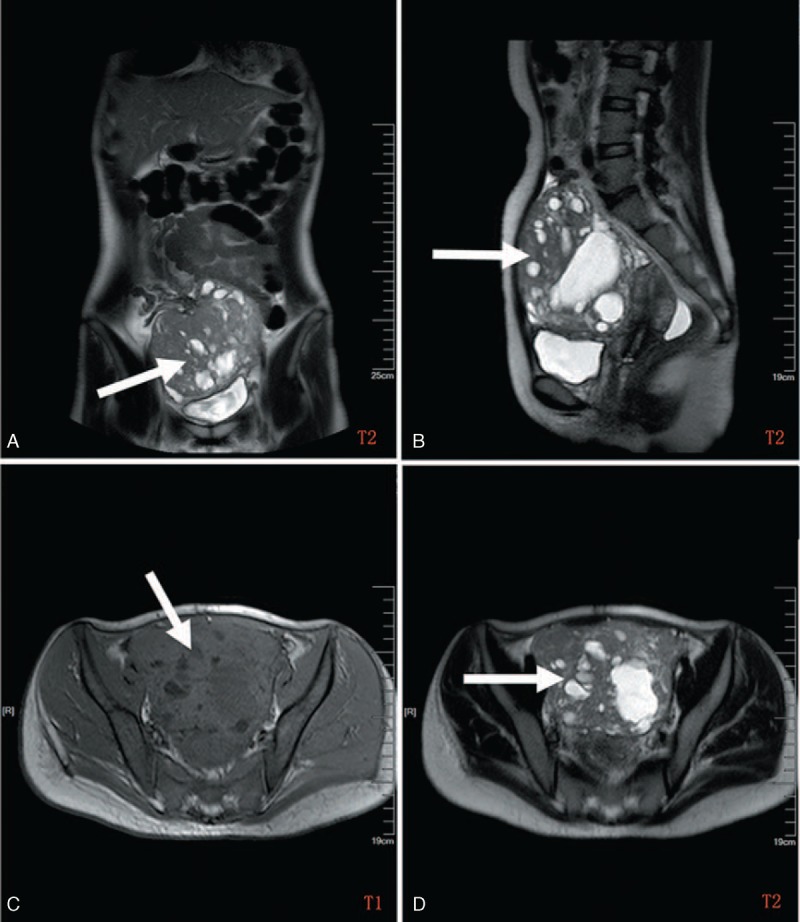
Abdominal computed tomography images (case 1): coronal (A), sagittal (B), and axial (C and D) reconstructions. Computed tomography of the abdomen showed an ill-defined multilocular low-density mass measuring 13 × 8 × 7 cm in the pelvis and lower abdomen.
